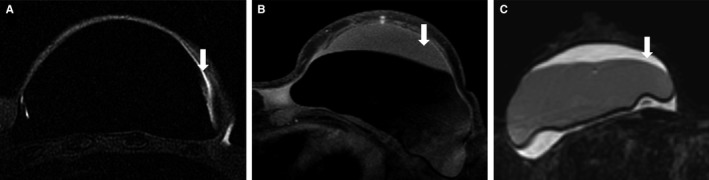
Representative cases on axial silicone‐suppressed magnetic resonance imaging (MRI) demonstrate the difference between physiologic fluid (white arrow) (A) and peri‐implant fluid collections (white arrow) (B and C)
